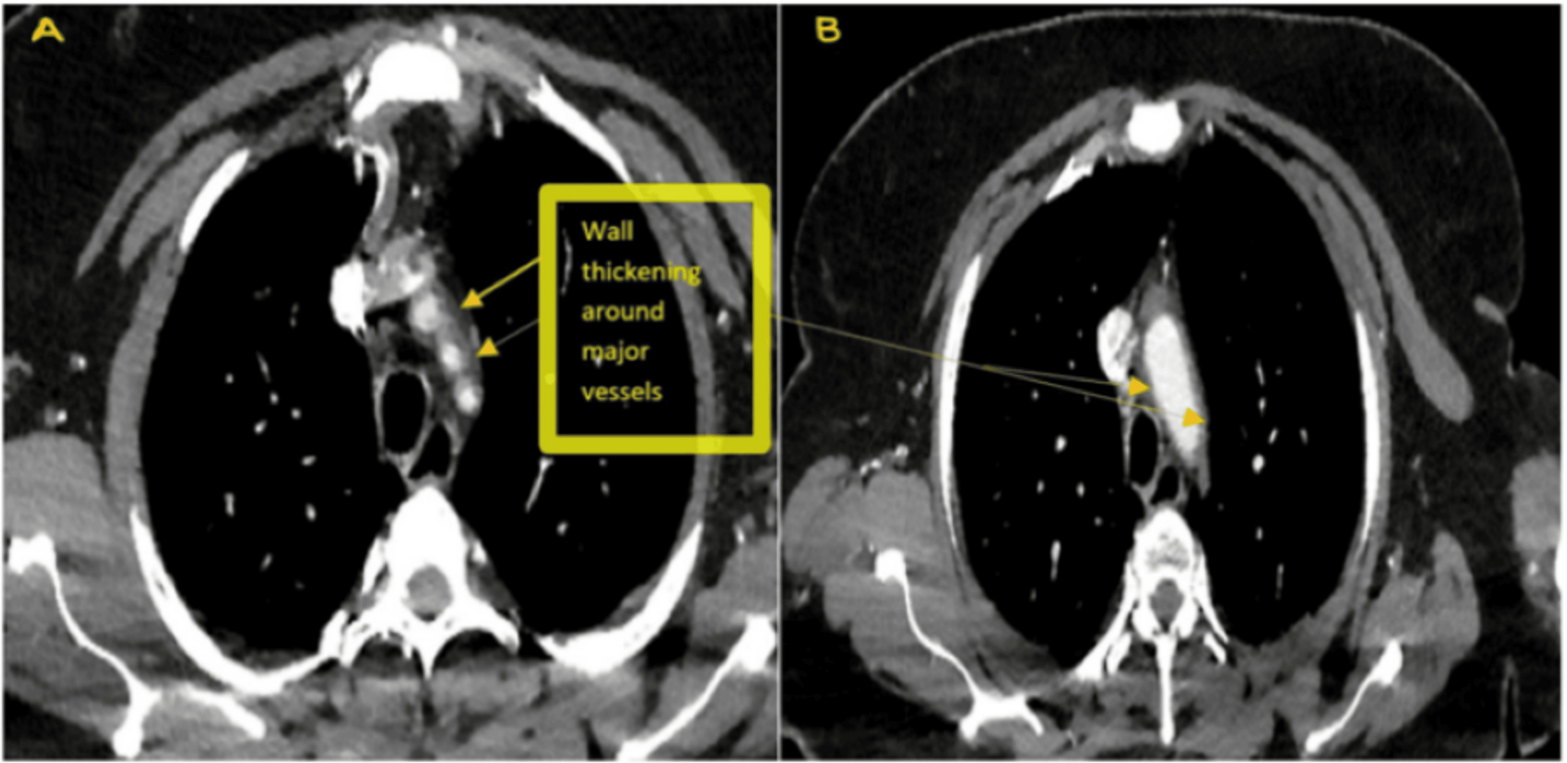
Initial CT angiography showing wall thickening around the major vessels
